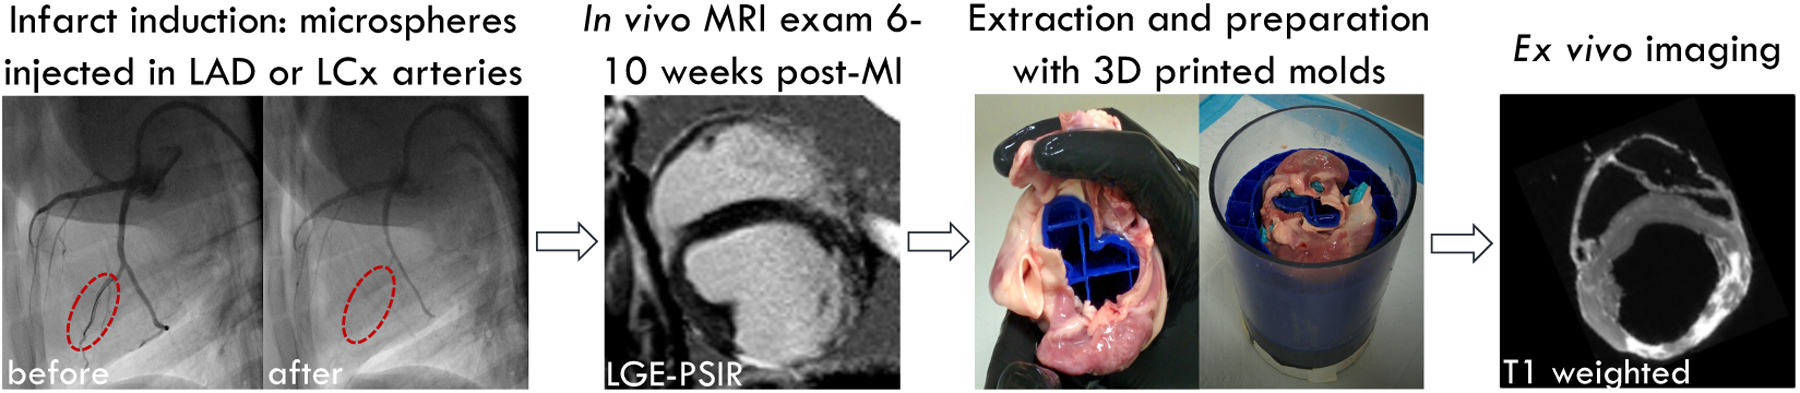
Experimental procedure: infarct induction and MR imaging
